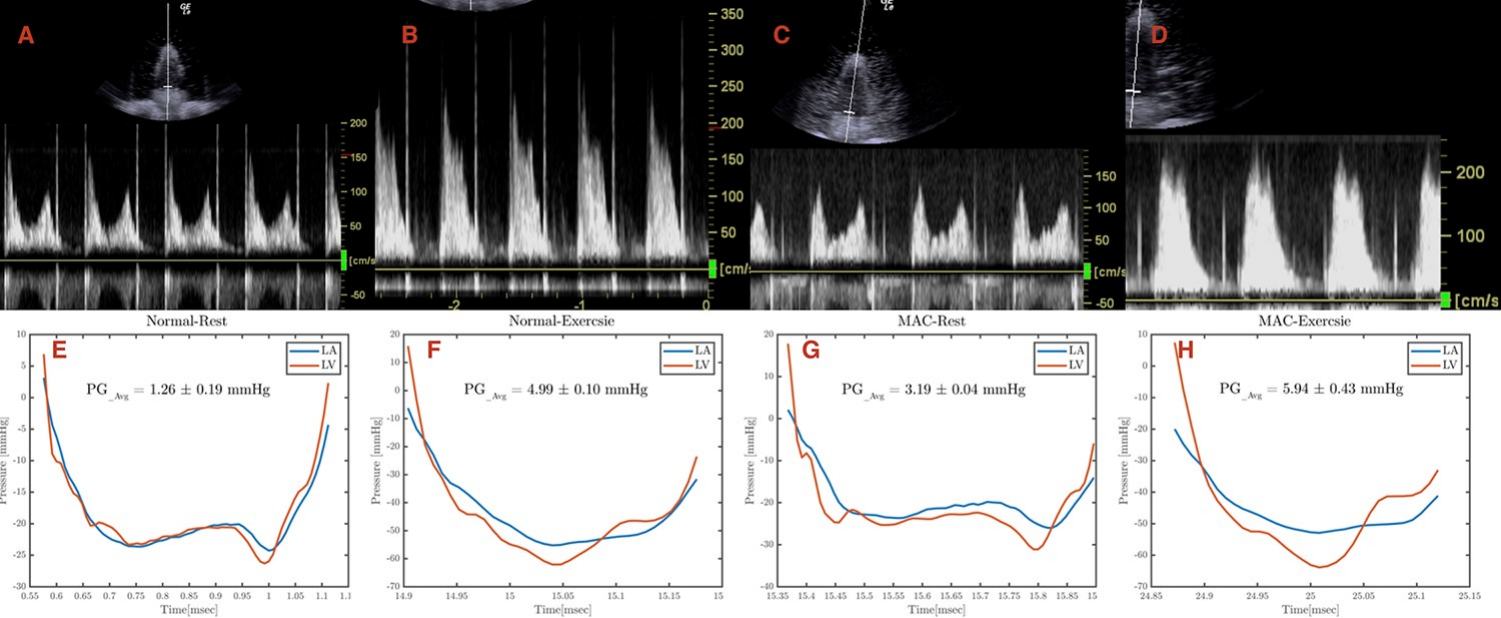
Echocardiographic and catheter assessment of pressure gradients. Continuous wave Doppler measurements of each experimental condition: (A) normal valve at rest, (B) normal valve during exercise, (C) mitral annular calcification (MAC) valve at rest, and (D) MAC valve during exercise. Catheter based pressure measurements of each experimental condition: (E) normal valve at rest, (F) normal valve during exercise, (G) mitral annular calcification (MAC) valve at rest, and (H) MAC valve during exercise.
